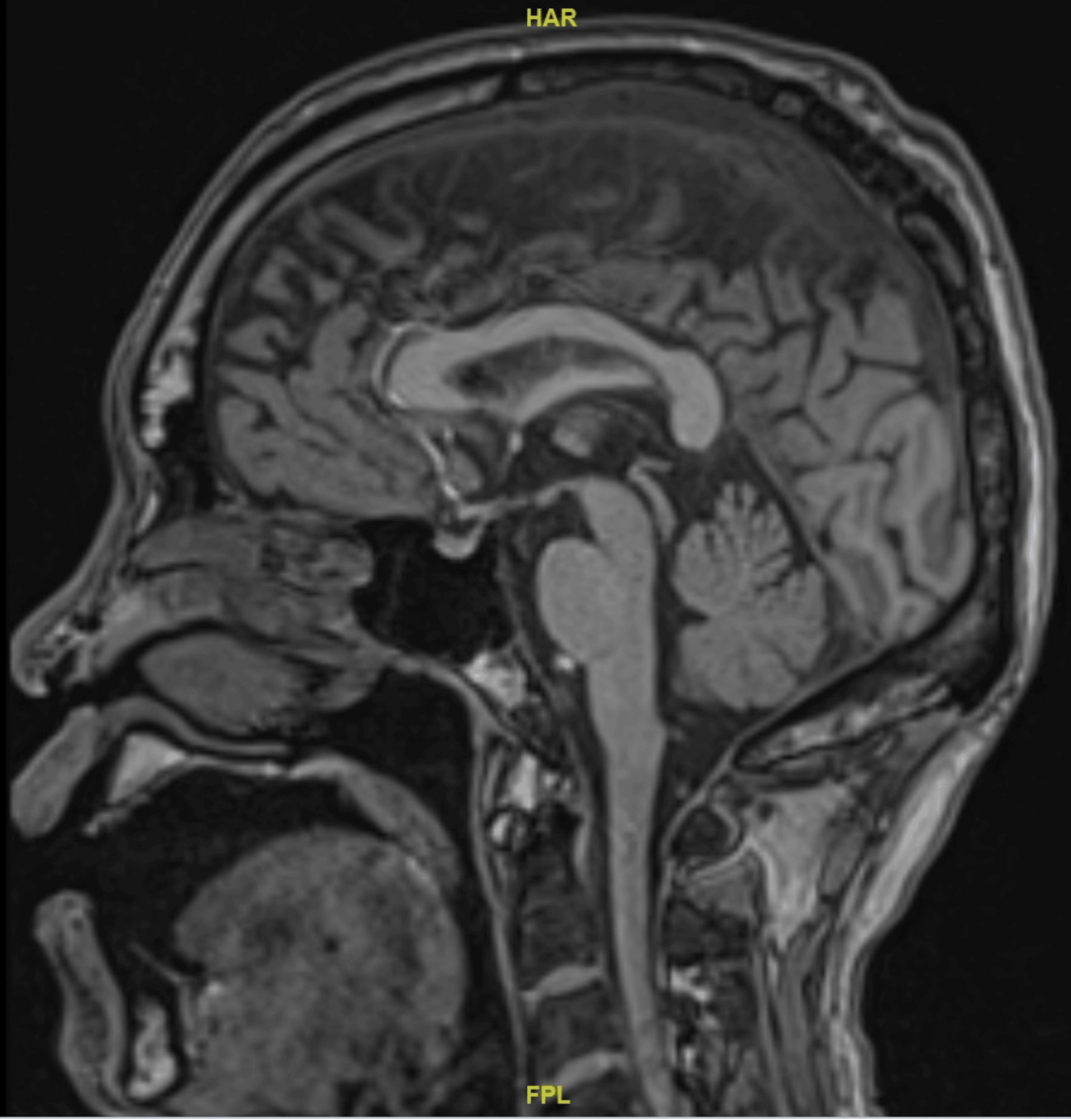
Non-contrast magnetic resonance imaging of the patient's brain, mid sagittal section Permission to use the above brain image for the purpose of this case report was obtained from Ng Teng Fong General Hospital.
